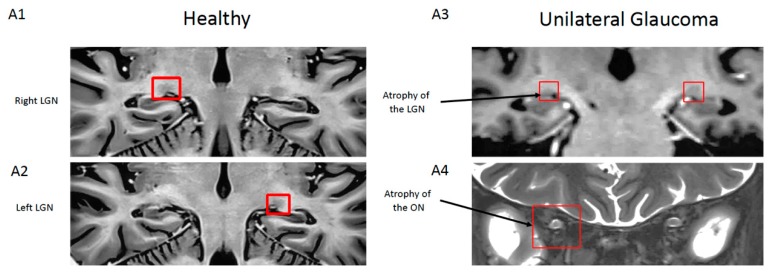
Magnetic resonance imaging (MRI) images of a coronal section of the brain of a healthy control and a unilateral glaucoma patient. Red squares localize the lateral geniculate nucleus (LGN) and optic nerve. MRI images from the patient show unilateral hyperintensity and atrophy of the right optic nerve (ON) and associated ipsilateral atrophy of the lateral geniculate nucleus compared to the healthy subject (from Dr. Habas Neuroimagerie XV-XX hospital; 3T SIEMENS Skyra MRI). (A1,2) Coronal contrast-inverted short-TI inversion-recovery (STIR) images of LGN. (A3) Coronal T1 weighted gradient-echo (MPRAGE) images of LGN. (A4) Coronal STIR image passing through the intraconal optic nerve.
